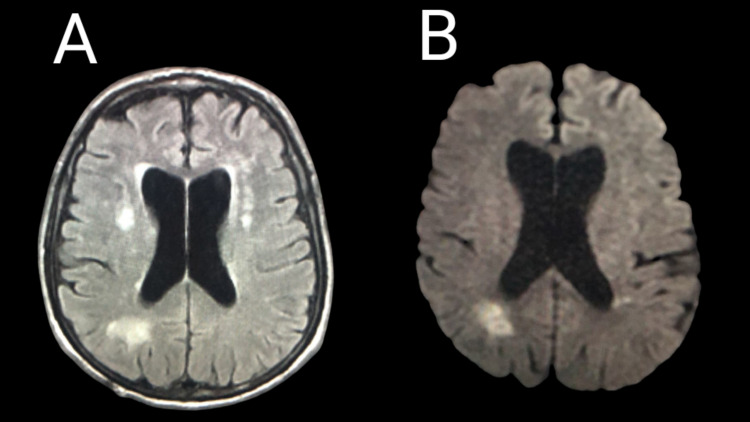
MRI of the brain at the level of the lateral ventricles. A: axial T2-FLAIR imaging showing multiple bilateral and peri-ventricular cortico-subcortical foci of high-intensity signal. B: axial DWI showing restriction of diffusion. DWI: diffusion-weighted imaging; MRI: magnetic resonance imaging; T2-FLAIR: T2-weighted fluid-attenuated inversion recovery
